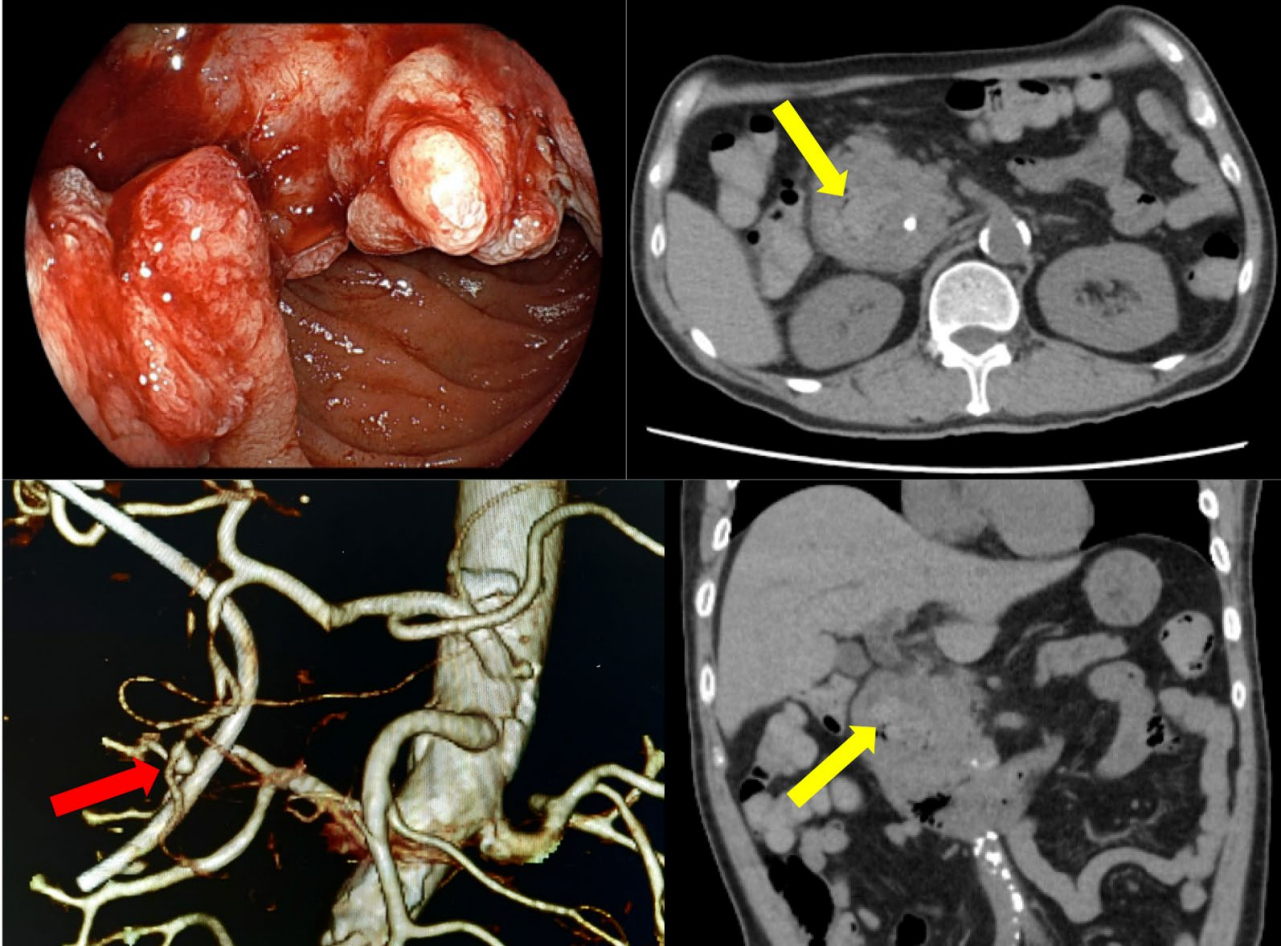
Findings of Emergency Endoscopy and Computed Tomography (CT), 3D-CT Angio: active hemorrhage from the duodenal tumor was observed on emergency endoscopy. A high-density region (yellow arrow) in the lumen of the lesion, suggesting an intraluminal hemorrhage and pseudoaneurysm formation (red arrow) in the gastroduodenal artery (GDA) branch (anterior superior pancreaticoduodenal artery) on CT and 3D-CT
